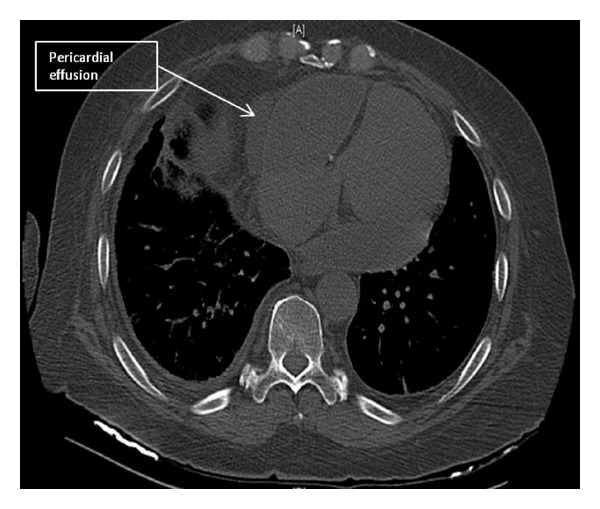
Axial noncontrast CT image of the thorax showing the heart with pericardial effusion.
